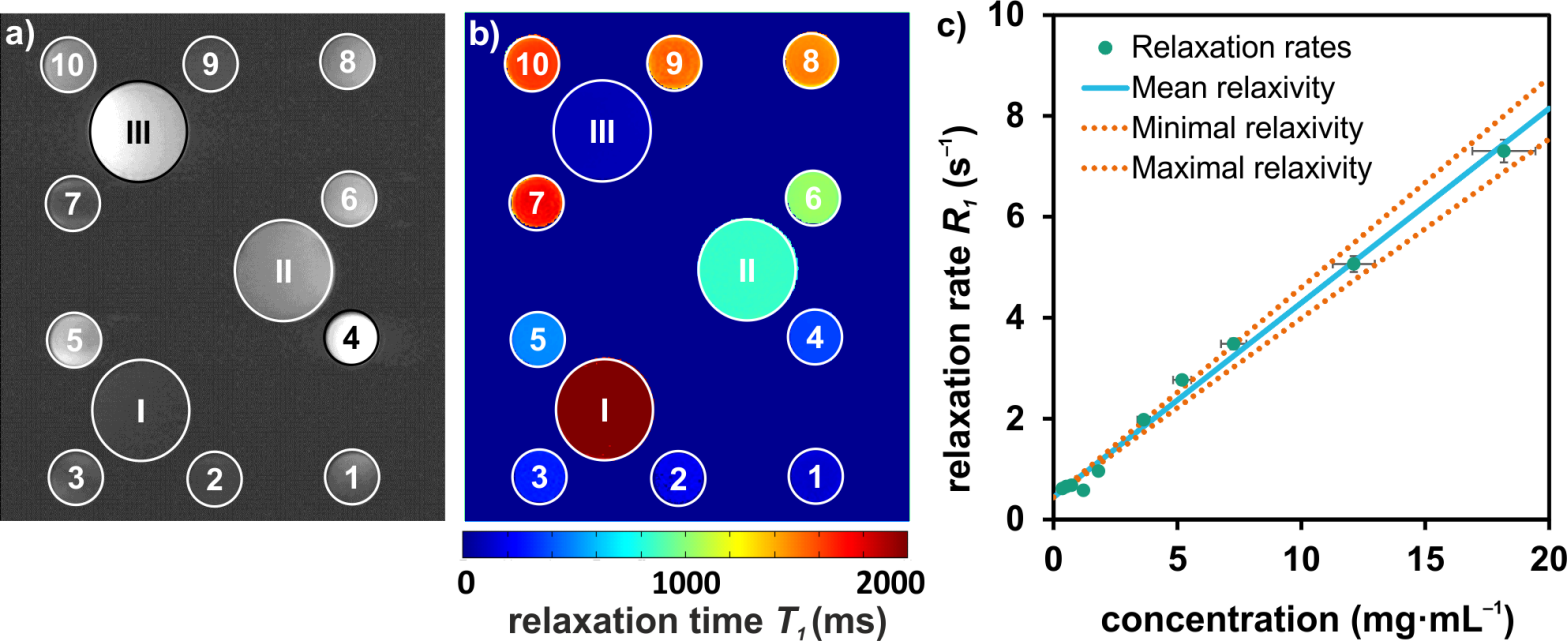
a) *T*_1_-weighted MR image of the CaF_2_:(Tb^3+^,Gd^3+^) NPs with different concentrations in the range from 0.4 to 18.2 mg·mL^−1^ (1–10). I–III are reference samples of water and two concentrations of Magnevist. The different concentrations result in observable differences of the signal intensities. In b) the following *T*_1_-map with a corresponding color range from 0 to 2000 ms is shown. c) The relaxivity (*r* = 0.385 ± 0.030 mL·mg^−1^·s^−1^) arises from the slope by plotting the relaxation rates over the concentrations. The errors of the relaxivity are given by the minimal and maximal slope.
